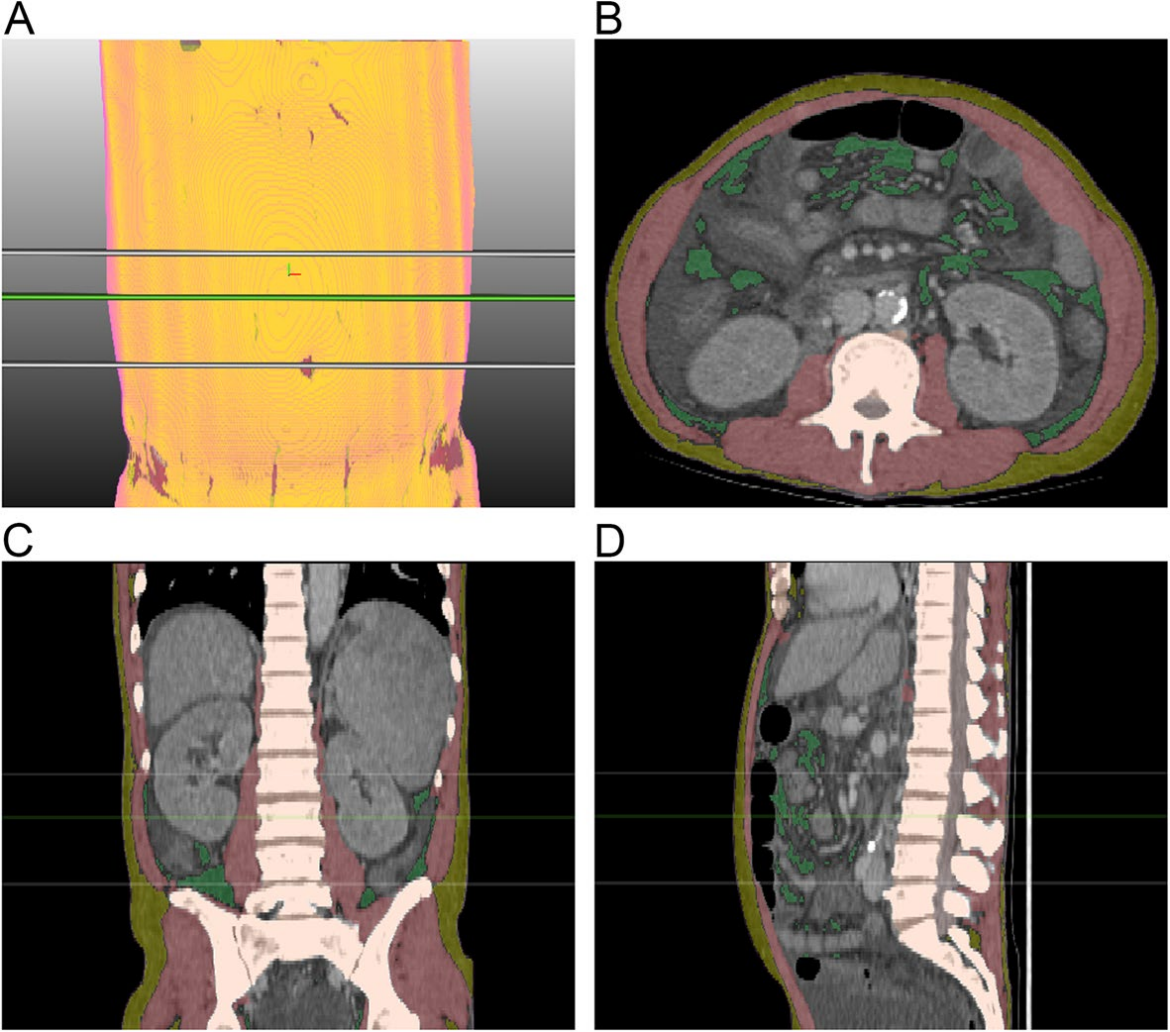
Representative three-dimensional (3D) reformatted (A) and cross-sectional (B-D) CT images of 3D U-Net that automatically segments CT images into a volumetric mask of seven body compartments in a 53-year-old male patient who underwent LT (body mass index, 26.2 kg/m.^2^). The overlapping lines represent the waist (white lines), L3 level (green line); skin (pink), subcutaneous fat (yellow), skeletal muscle (brown), abdominal visceral fat (light green), bone (light beige), internal organs and ascites (light gray), and central nervous system (light pink)
